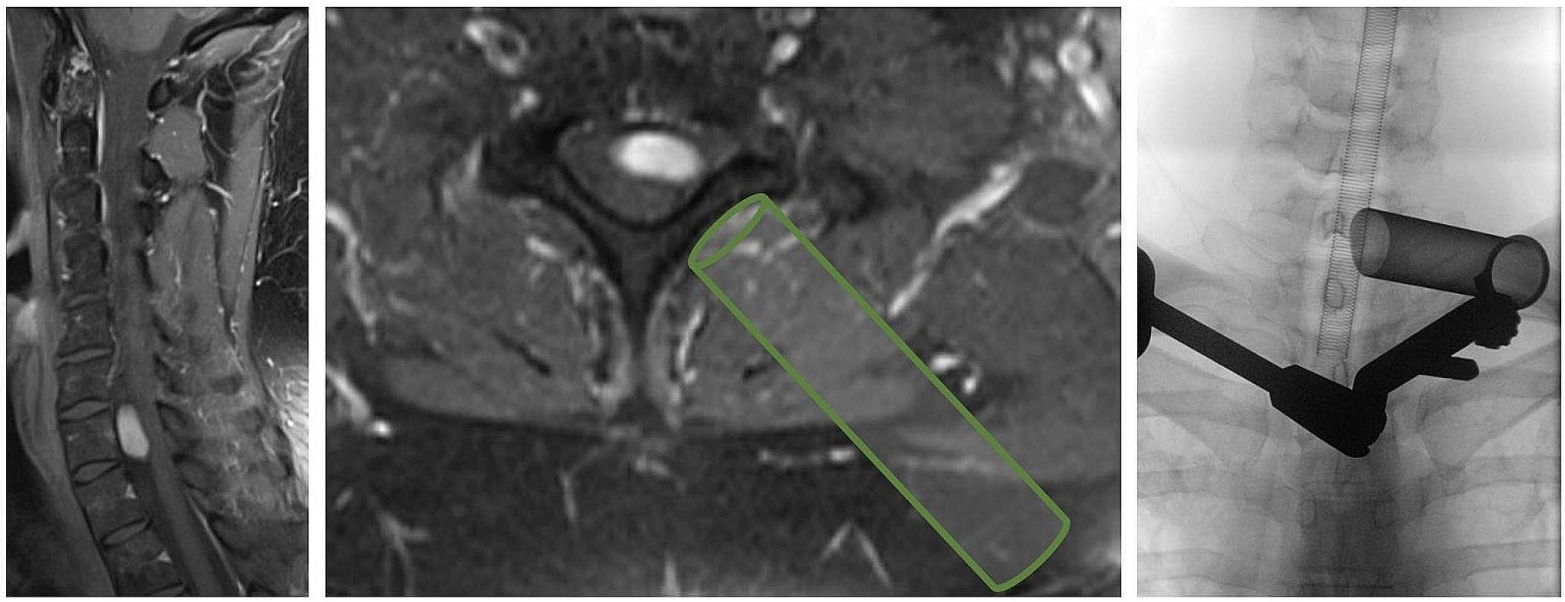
Exemplary case of an intraspinal intradural BNST anterior to the spinal cord at the level of C7 on contrast enhanced T1 weighed MRI (left). Axial MRI shows superimposed the minimally invasive tubular approach in green (middle). Intraoperative anterior-posterior X-ray of the tubular retractor placement (right)
